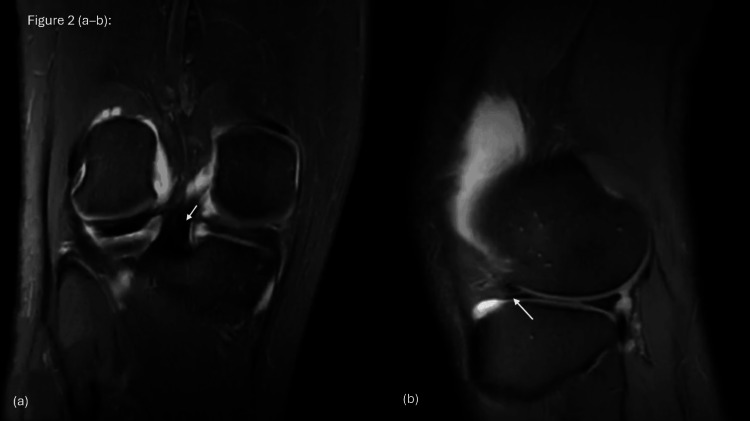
MRI findings showing (a) coronal T2-weighted FSE FS images demonstrating a medial meniscus tear (white arrow), and (b) sagittal T2-weighted FSE FS images showing a lateral meniscus anterior horn tear (white arrow). FSE FS: fast spin-echo fat-suppressed
